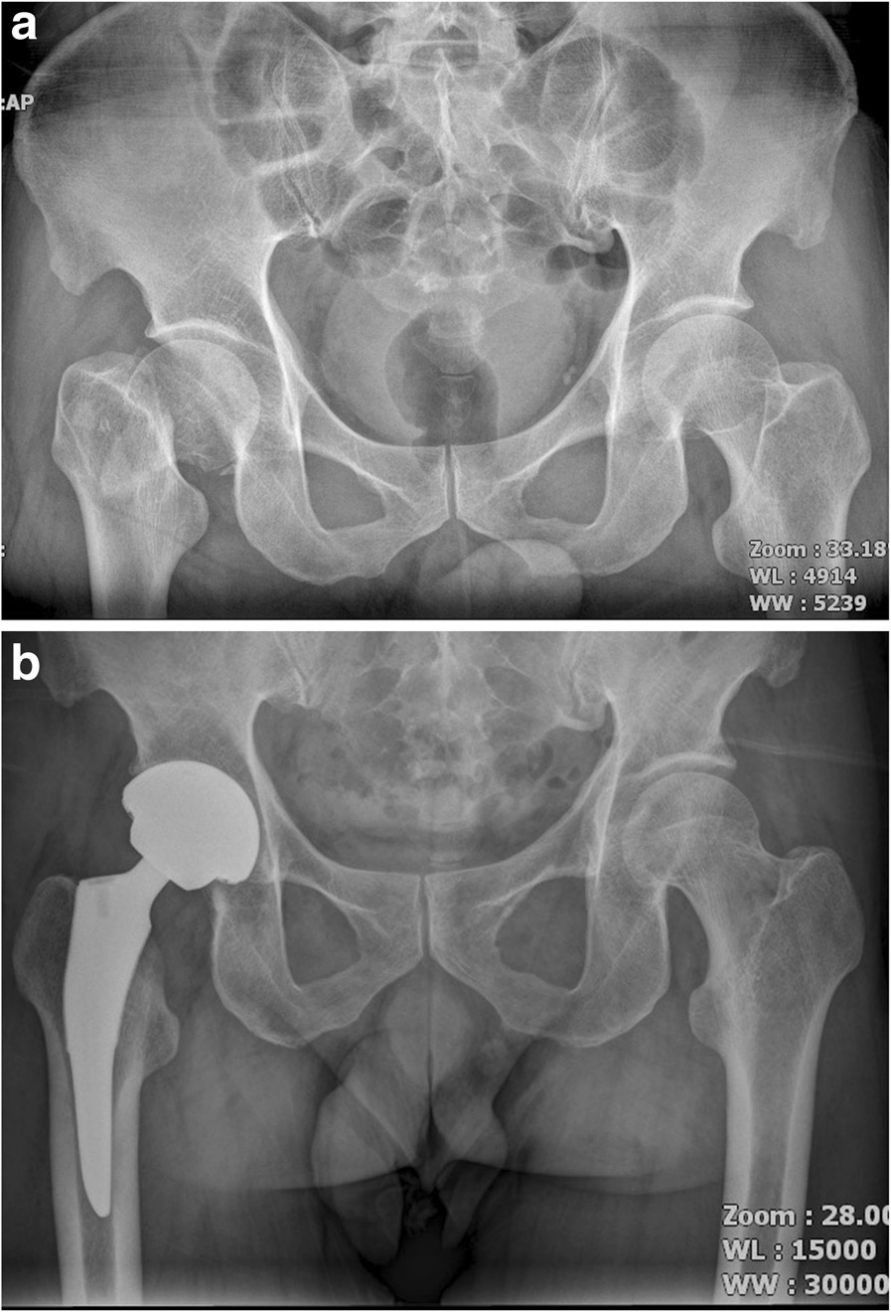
a A preoperative radiograph of the hip of a 73-year-old man who had right femoral head neck fracture. b A radiograph at 40 months after implantation of short cementless femoral stem showed solid fixation in a satisfactory position
